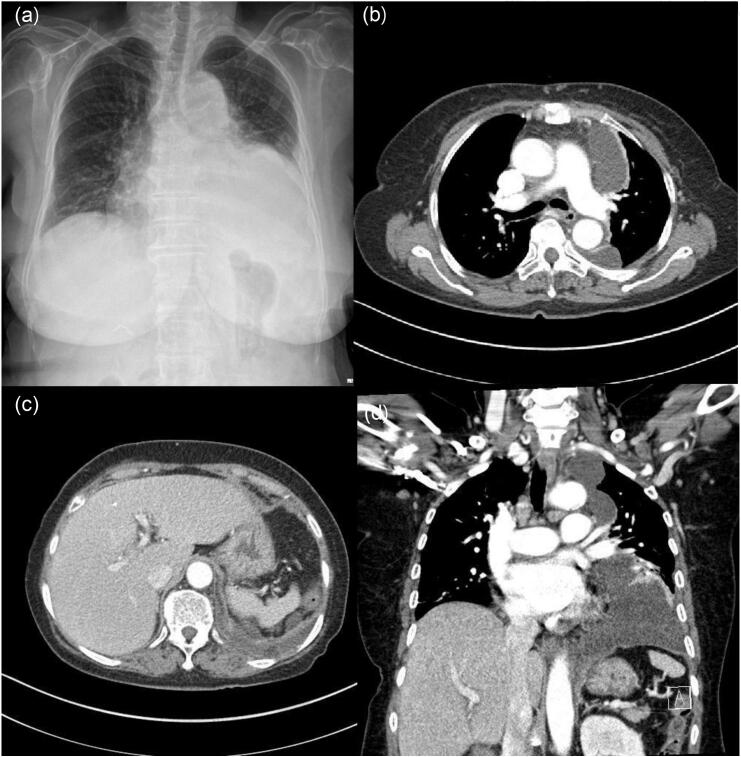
(a) Chest radiograph shows increase in amount of left pleural effusion with consolidation and atelectasis in left lower zone. (b–d) show multilobulated pleural effusion in left hemithorax and left diaphragmatic defect.
